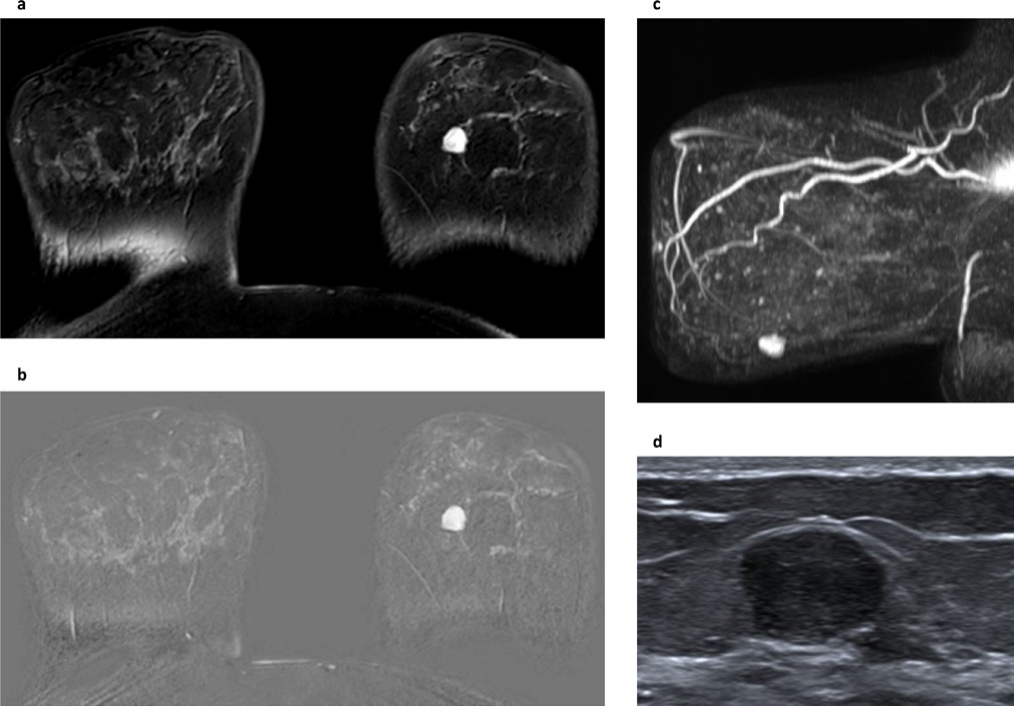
Fibroadenoma. This early post-contrast dynamic image (fat-suppressed T1W) (a) shows a biopsy-confirmed fibroadenoma in the left breast. It appears predominantly well circumscribed, but its high signal on this sequence represents avid enhancement which at this (early) time point demonstrates a fast initial enhancement curve. The equivalent subtracted image (b) contrasts the intense gadolinium uptake within the fibroadenoma with the much less intense normal bilateral background parenchymal enhancement and also shows that the internal enhancement pattern is heterogenous. c is the sagittal maximum intensity projection of the summated subtracted slices for the left breast and shows that the fibroadenoma is solitary and dominant within the breast. d is an image from the ultrasound correlation for the lesion.
